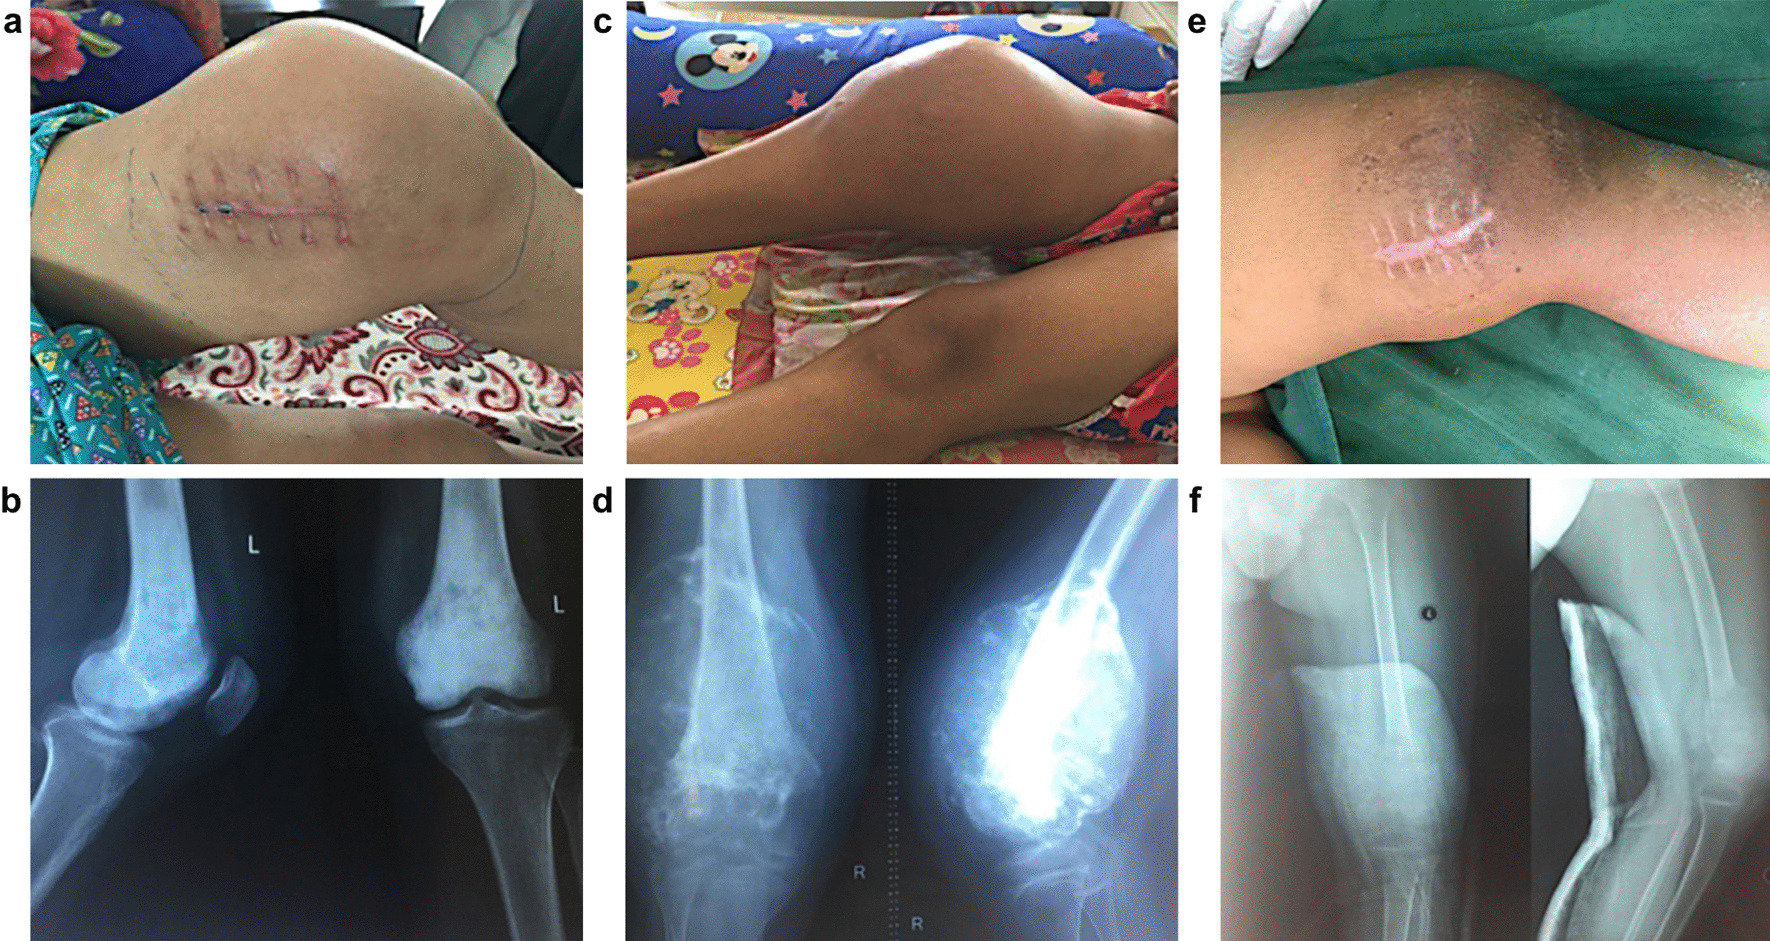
Clinical images and X-rays of patients 1–3. a and b Patient 1 with osteosarcoma of the left distal femur and corresponding X-ray; c and d Patient 2 with osteosarcoma of the right distal femur and corresponding X-ray; e and f Patient 3 with osteosarcoma of the left distal femur and corresponding X-ray
